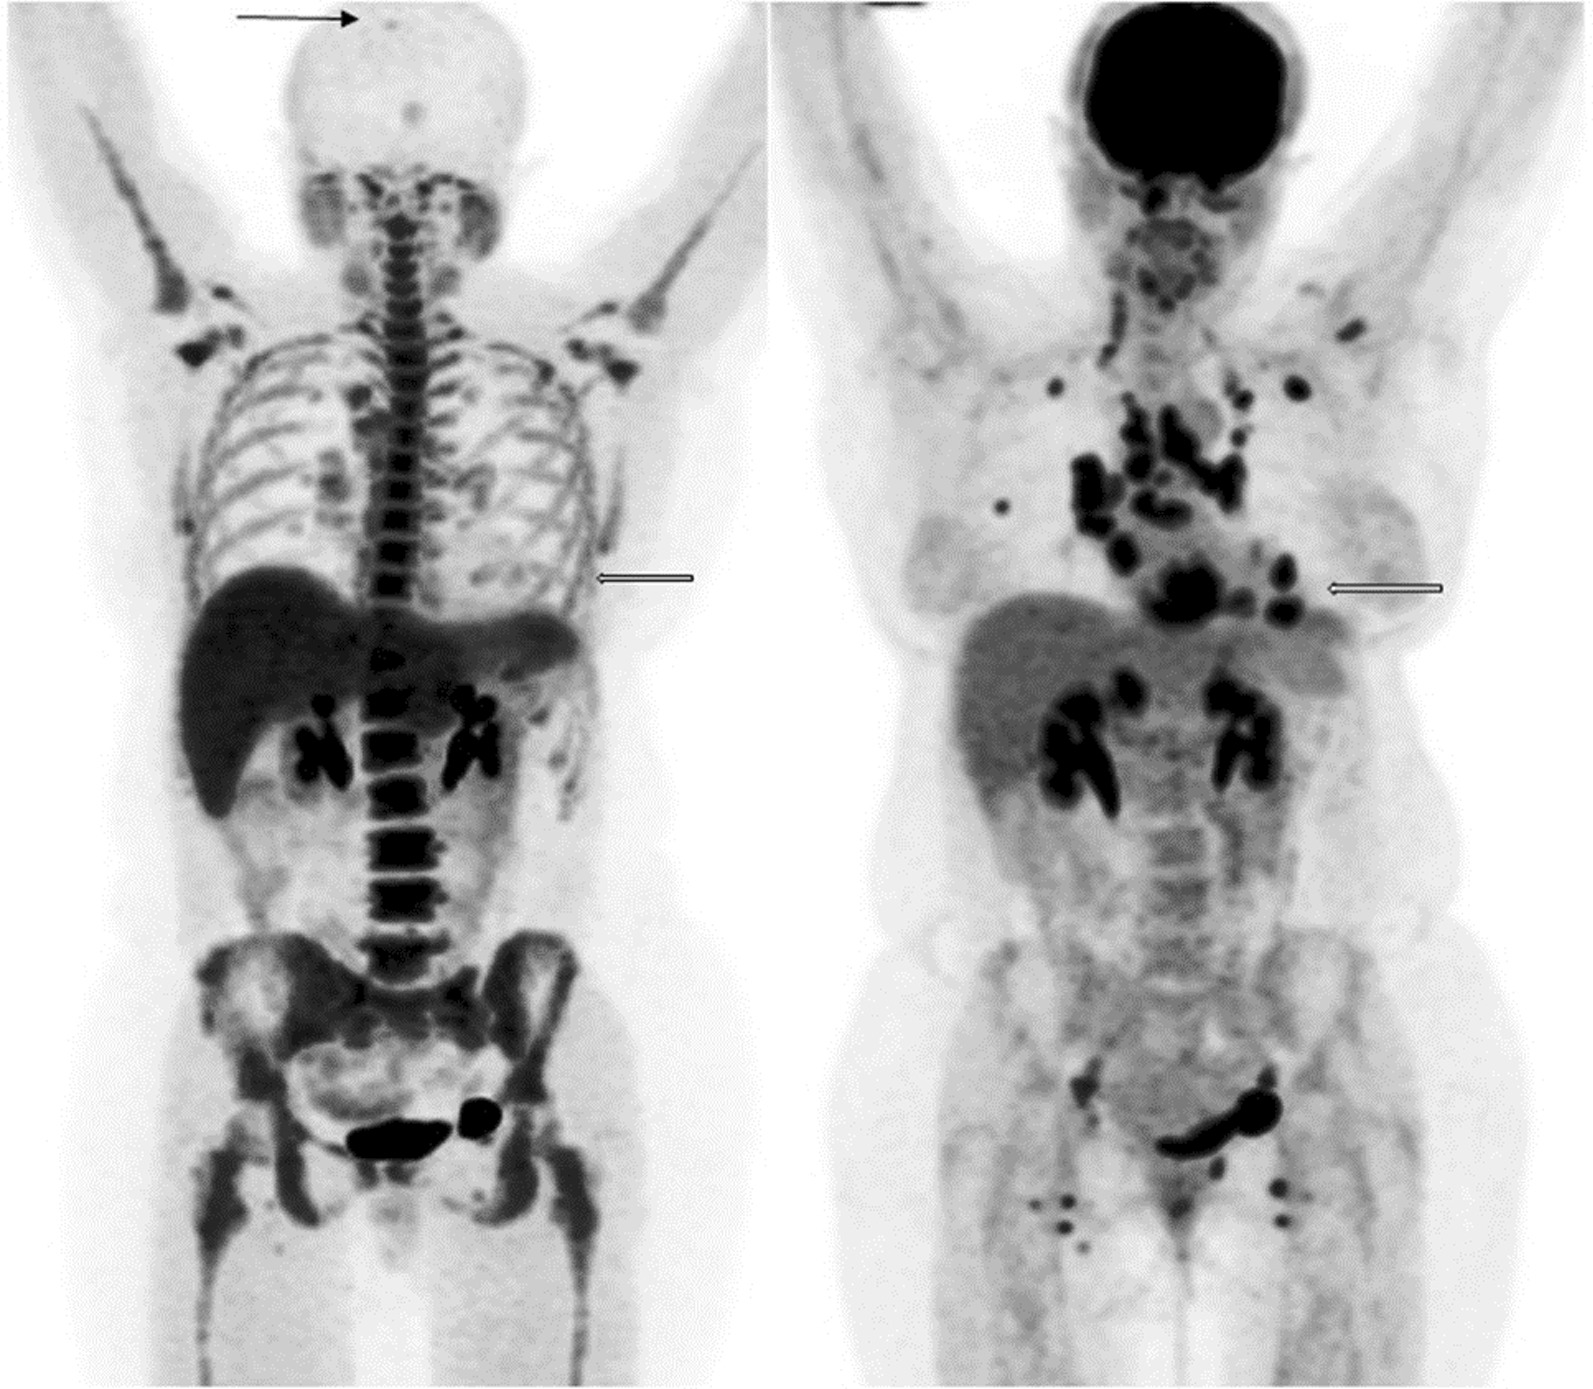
Whole-body maximum intensity projections of FLT-PET (left) and FDG-PET (right) studies in the same subject (subject #4) with evidence of sarcoidosis, including cardiac and neural involvement (arrows)
